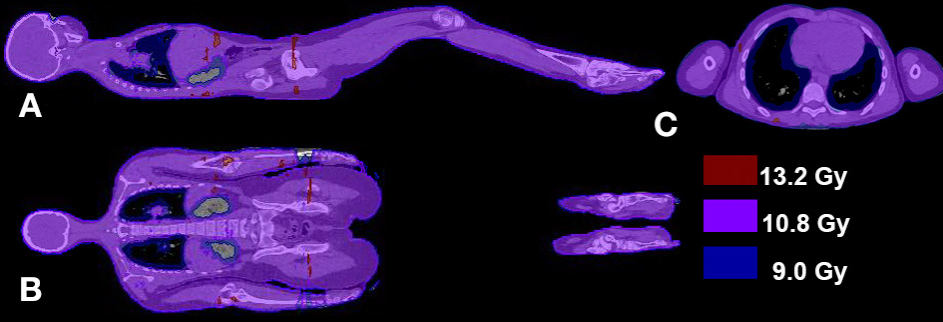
CT-planned VMAT total body irradiation technique dose distribution. Computed tomography (CT)-planned volumetric-modulated arc therapy (VMAT) total body irradiation technique dose distribution for a 12 Gy prescription dose in the sagittal (A), coronal (B), and transversal view (C). The isofill-depicted dose levels are 75% (9 Gy; blue), 90% (10.8 Gy; purple), and 110% (13.2 Gy; red) of the prescription dose.
